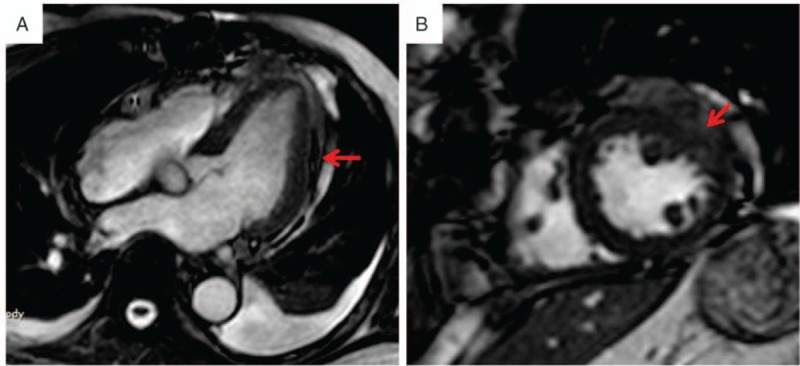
Postoperative cardiac magnetic resonance images, (A) long axis view of cardiac magnetic resonance after surgery (red arrow: repair of the injury region), (B) short axis view of cardiac magnetic resonance after surgery (red arrow: repair of the injury region).
